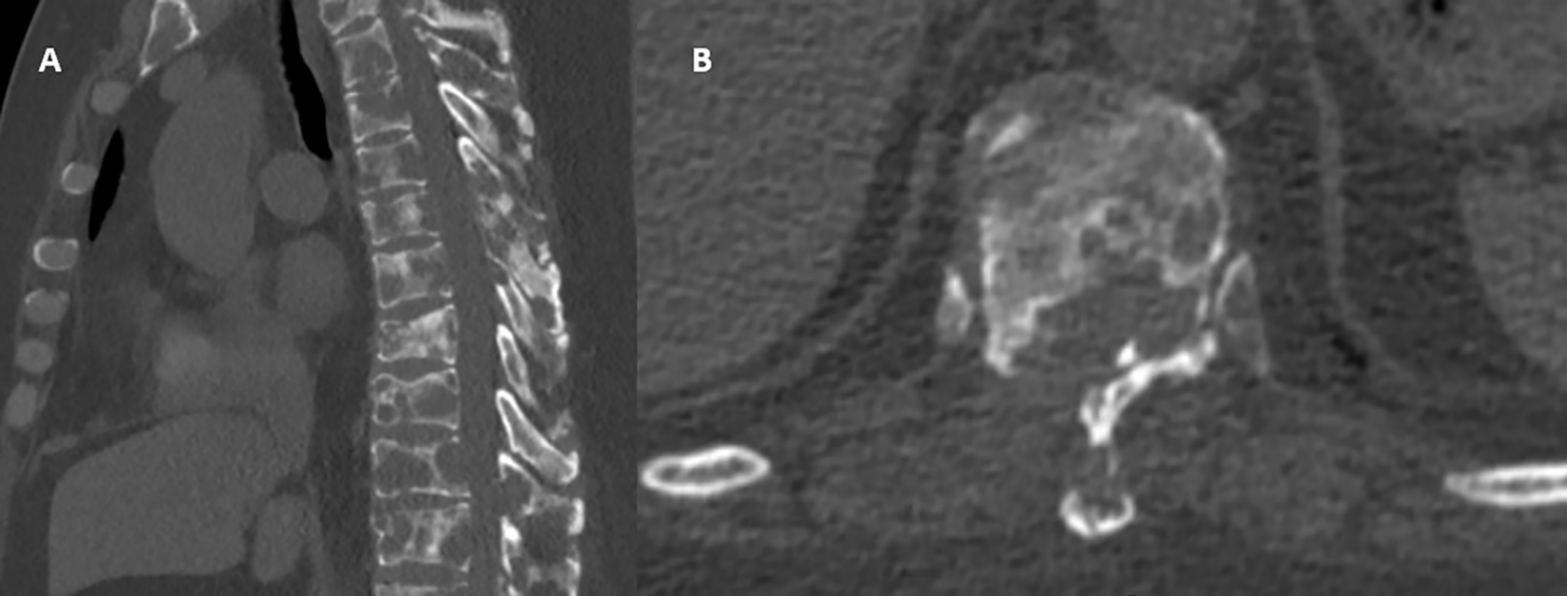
Postoperative CT imaging: (A) Sagittal view: confirmed decompression of the spinal canal at the Th10-Th11 level with partial removal of destructive masses and partial restoration of canal patency. Metastatic lesions persist within the vertebral bodies; however, compression is visually reduced due to surgical debridement. (B) Axial view: deformed Th10 vertebra with marked osteolytic destruction of the vertebral body and posterior wall; the spinal canal appears reconstructed. Paravertebral soft tissue components are present without evidence of volume progression.
